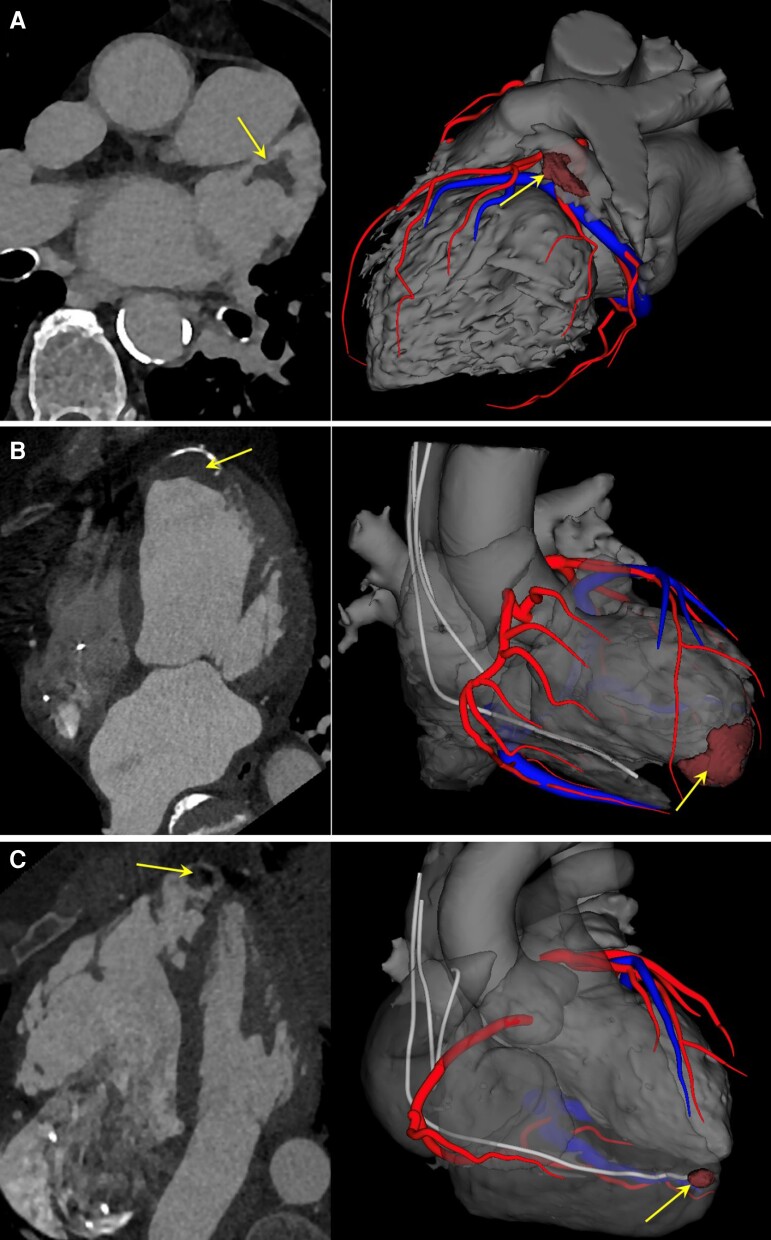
Examples of intra-cardiac thrombi on CT scan in patients referred for VT ablation. (*A*) Thrombus located in the left atrial appendage in a patient referred for VT ablation with associated history of atrial fibrillation. (*B*) Thrombus located at left ventricular apex in a patient with post-infarction VT and calcified apical aneurysm. (*C*) Thrombus located at right ventricular apex in a patient with arrhythmogenic right ventricular cardiomyopathy. Left column: contrast-enhanced CT. Right column: 3D modelling via Inheart technology. Arrows indicate thrombus location.
